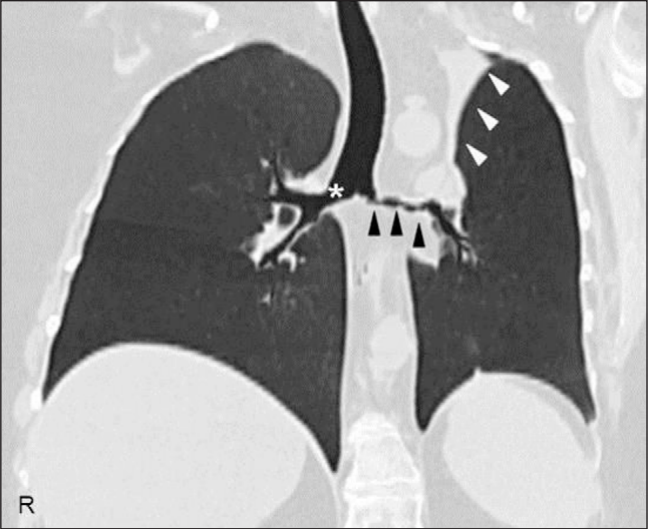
High-resolution computed tomography of the chest, coronal reconstruction, showing a marked wall thickening of the right main bronchus (asterisk), stenosis of the left main bronchus (black arrowheads), and segmental atelectasis in the upper lobe of the left lung (white arrowheads).
